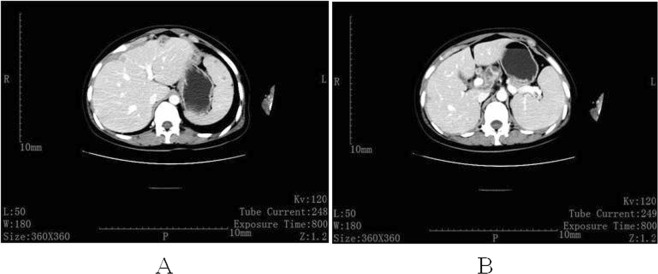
Abdominal enhanced CT of case 18. (A) Irregular thickness of the peritoneum and local nodular shape protruding to the liver surface; multiple nodules in the liver surface, with uneven enhancement and multiple local necrosis; larger in the spleen, with small circular low density and slight enhancement. (B) Multiple enlarged lymphoglandulae coeliacae coalescing partly and annular enhanced lesions, and low density shadow in the local liver.
